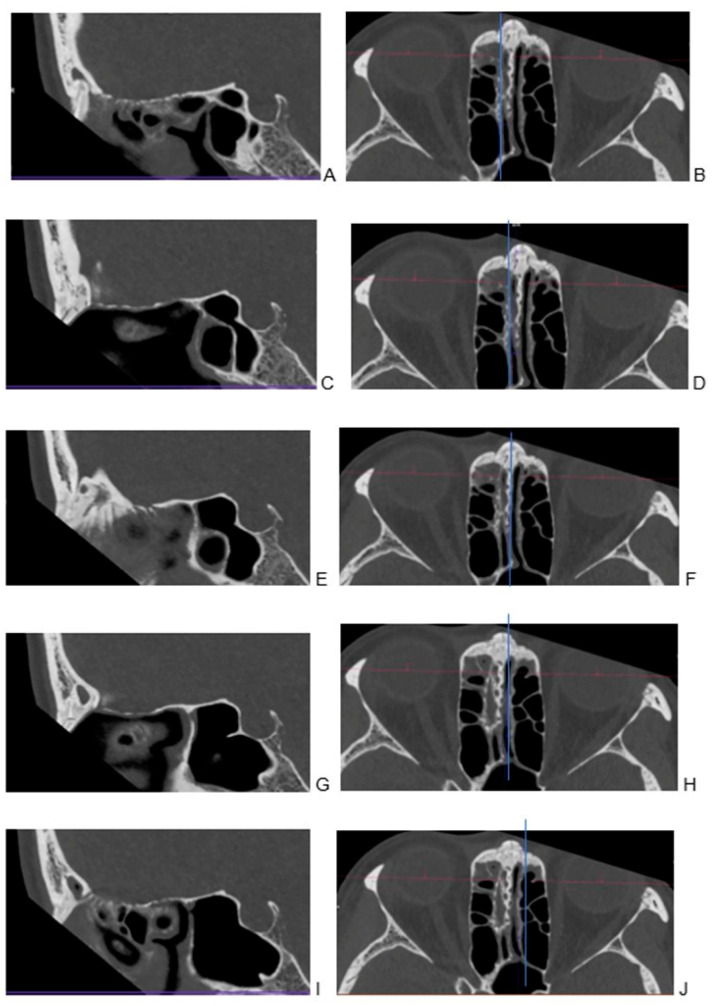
Visualization of cribriform plate in photon-counting computed tomography. Visualization of cribriform plate in photon-counting computed tomography. Examination in multiplanar reformation (A–J). To facilitate referencing specific layers in sagittal imaging with 0.2 mm, divided into several distinct regions for closer examination: (A) the right lateral part of the cribriform plate, showcasing its specific features and characteristics; (B) a corresponding transverse view; (C) moving towards the right side, the portion situated between the lateral and middle sections of the cribriform plate; (D) a corresponding transverse view; (E) transitioning to the sagittal plane with a focus on the middle part of the cribriform plate; (F) a corresponding transverse view accompanying the sagittal perspective to aid in comprehensive examination; (G) on the left side, a space located between the lateral and middle sections; (H) a corresponding transverse view provides a complementary angle; (I) the left lateral part of the cribriform plate; (J) a corresponding transverse view.
